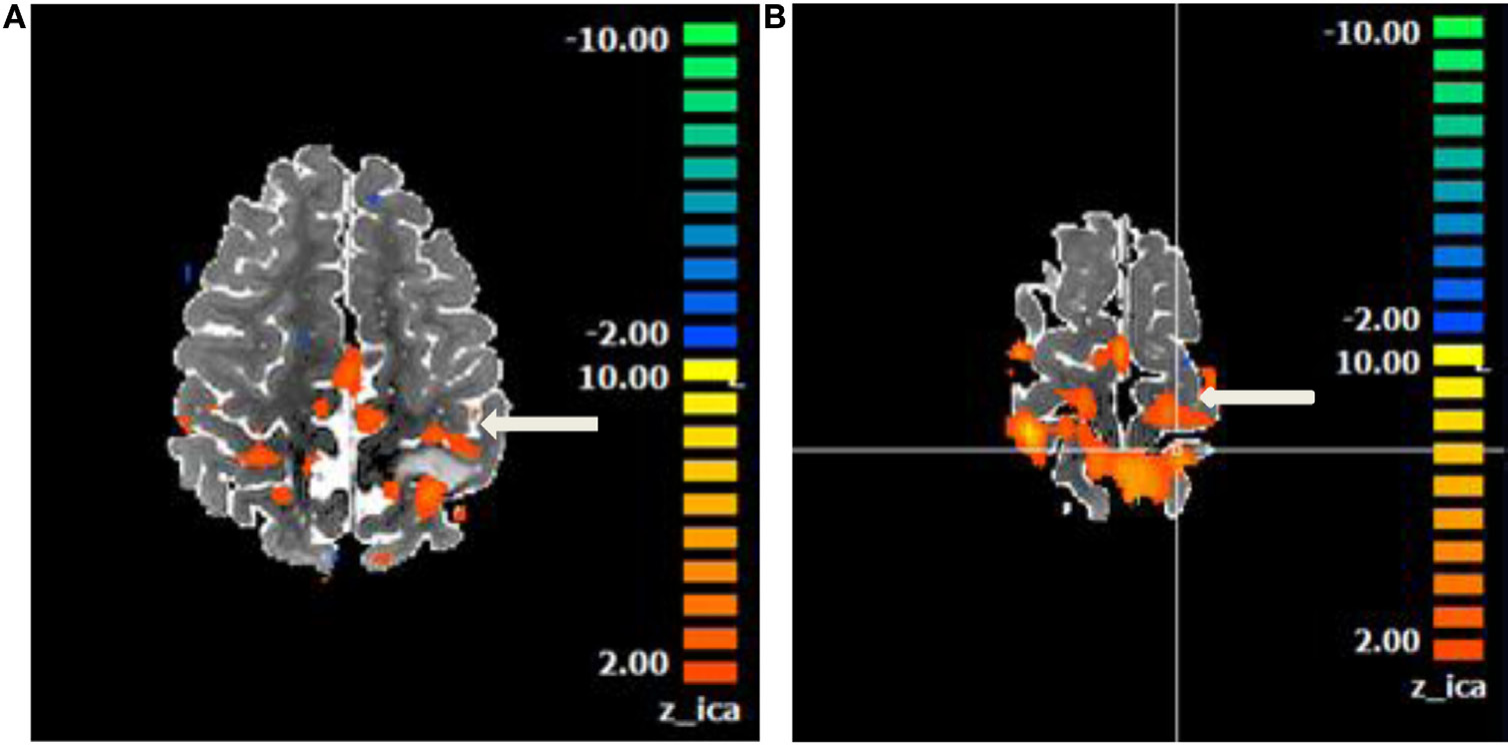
Motor network identified on a resting state functional magnetic resonance imaging in an anesthetized 6-year-old demonstrating eloquent cortex on both sides of a left postcentral gyrus glioma. Central sulcus is marked with white arrow. (A) Axial T2 image with overlaid motor network, in red, separated by T2 hyperintense glioma. (B) Axial T2 image with overlaid motor network on an image slice immediately superior to image (A). No copyright permissions were required to use these images.
